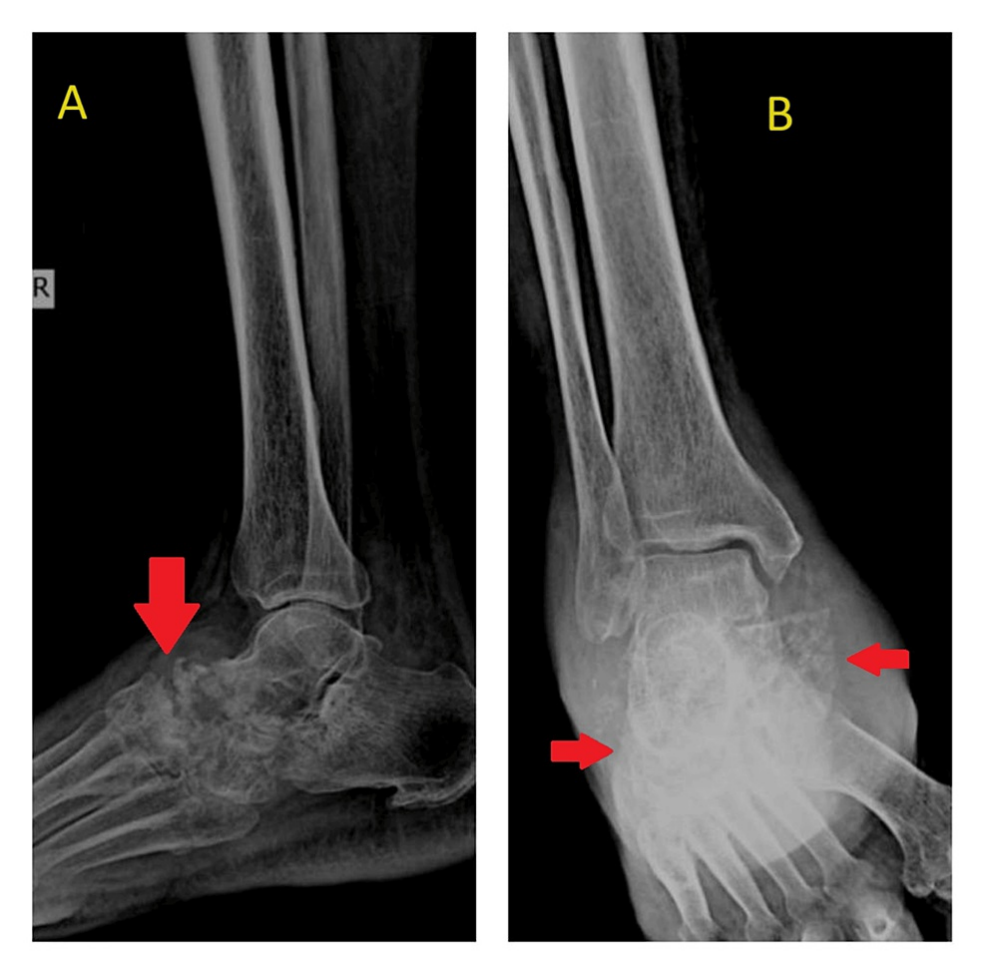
X-ray of right ankle lateral (A) and mortise (B) views showing osteomyelitis changes of the right intertarsal joints and tarsometatarsal joints.
